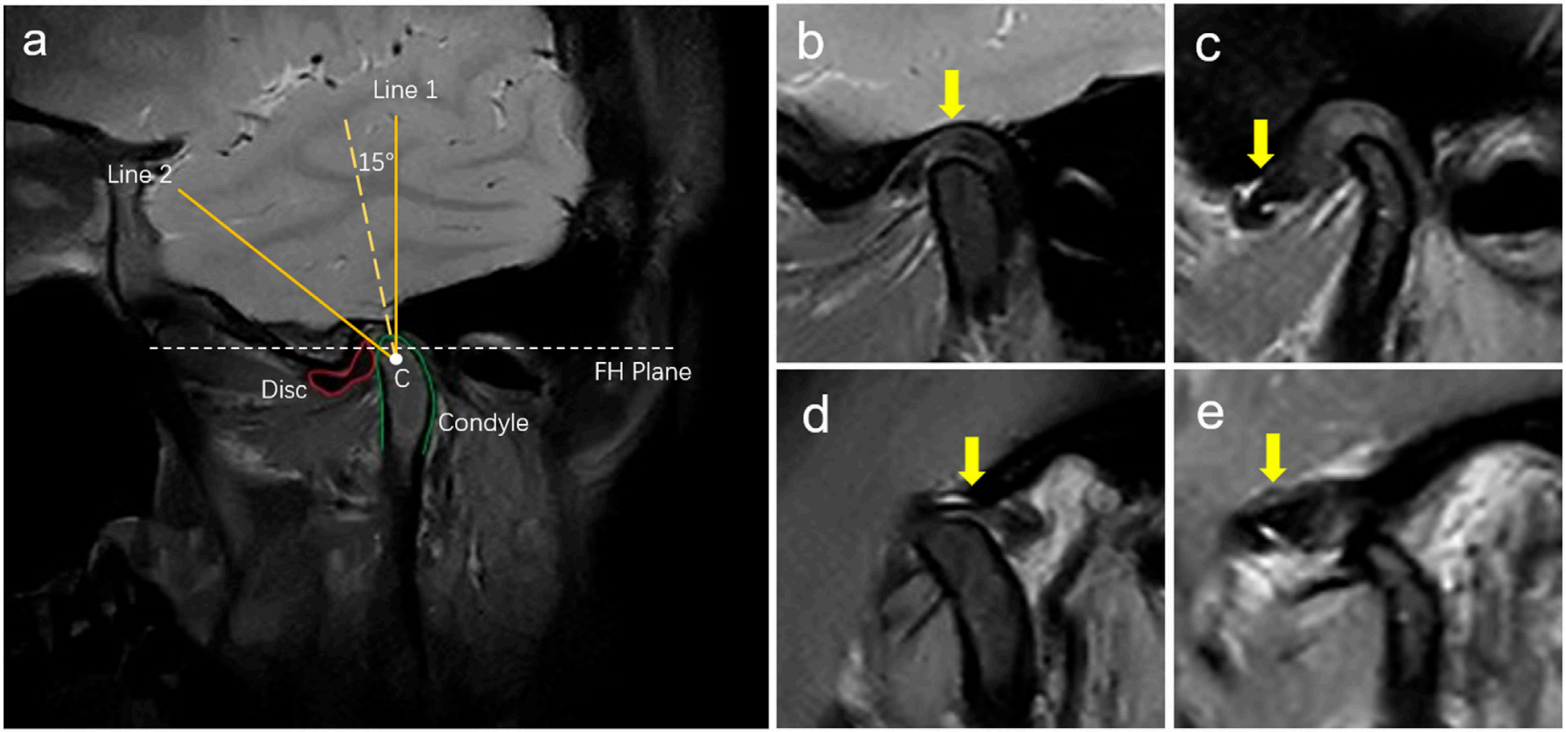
Diagnosis of disc displacements in MRIs. (A) MRI diagnostic method for TMJ ADD. C is the center of the condylar head; Line 1 is the line passing over the condylar apex and point C; Line 2 is the line passing over the posterior margin of the posterior band of the TMJ disc and point C; (B) N-CMP; (C) ADD-CMP; (D) N-OMP; (E) ADD-OMP. The arrow points to the location of the TMJ disc.
